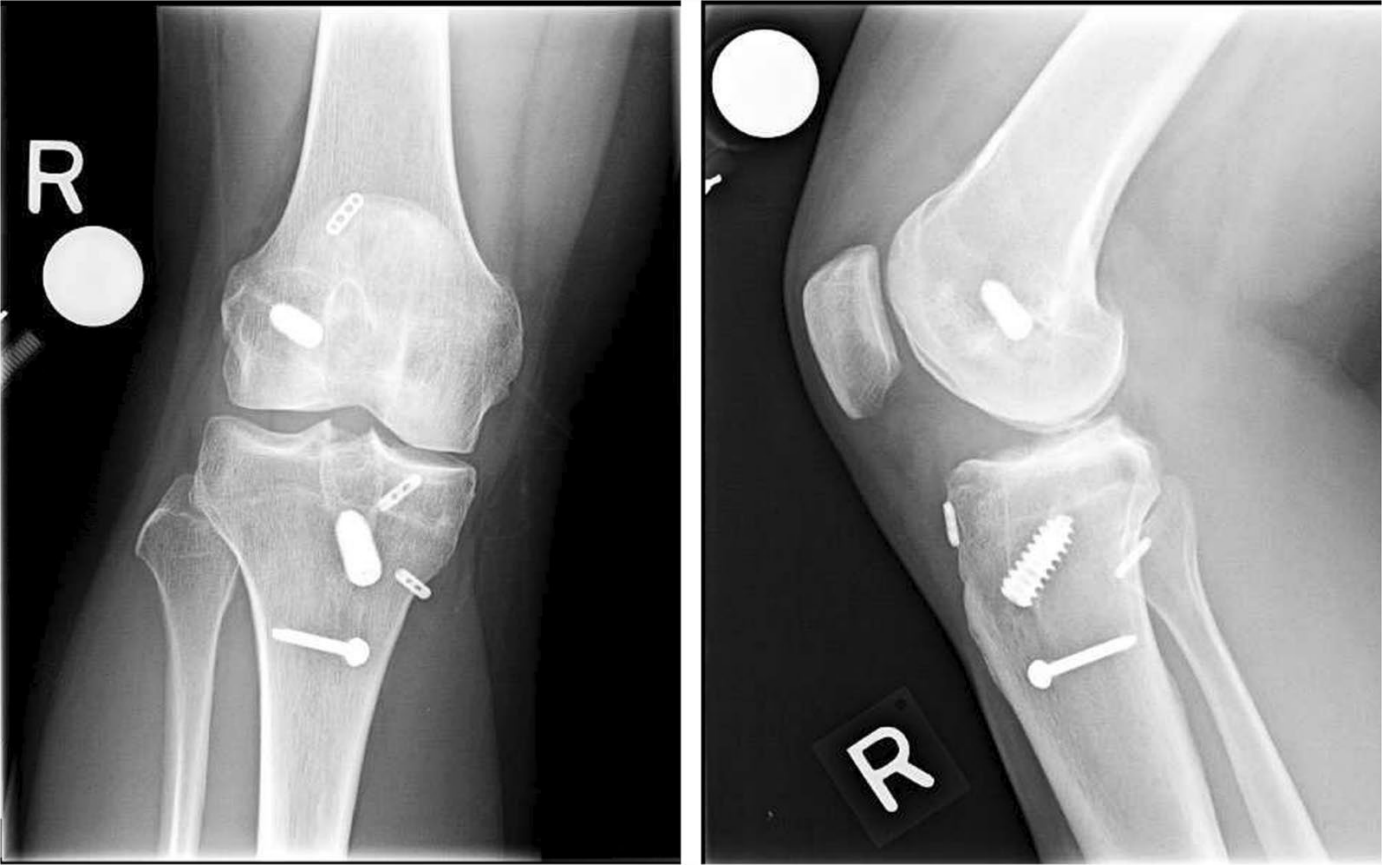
Knee X-rays with AP view (left) and lateral view (right) taken at 17 months following medial MAT and revision ACLR
